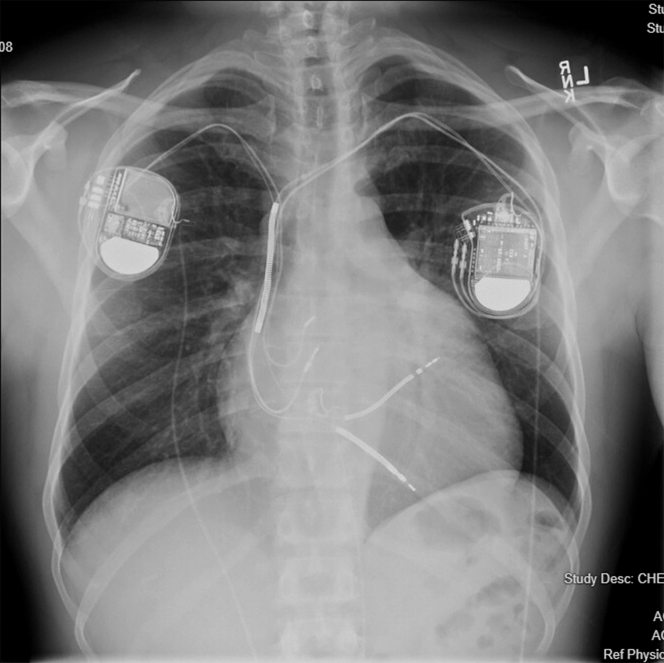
Chest Plain Film Original dual-chamber ICD (patient's left) was placed in 2002, which was complicated by lead fracture, venous occlusion, and abandonment. Replacement dual-chamber ICD (patient's right) was placed in 2017. Multiple leads residing in the central venous system resulted in chronic superior vena cava syndrome. ICD = implantable cardioverter-defibrillator.
